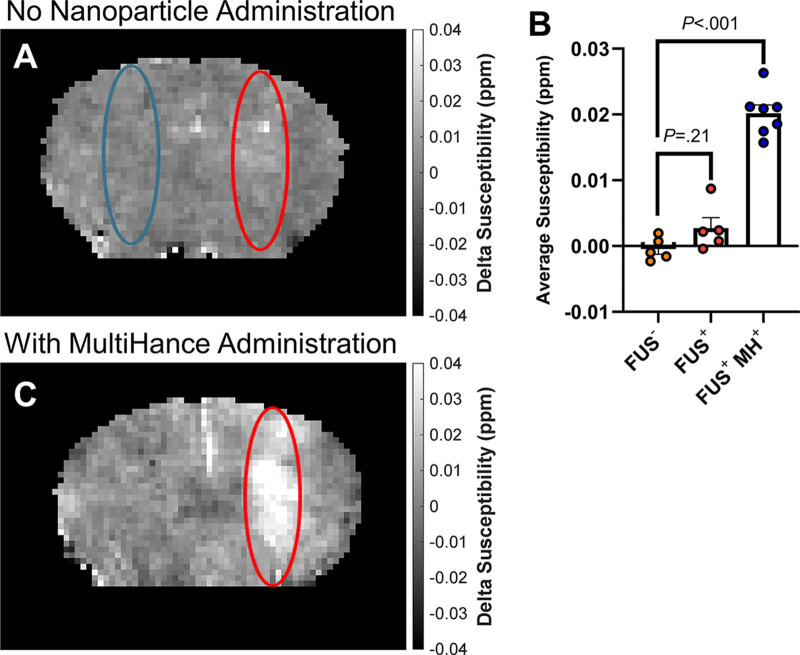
Effect of focused ultrasound (FUS)–mediated blood-brain barrier
